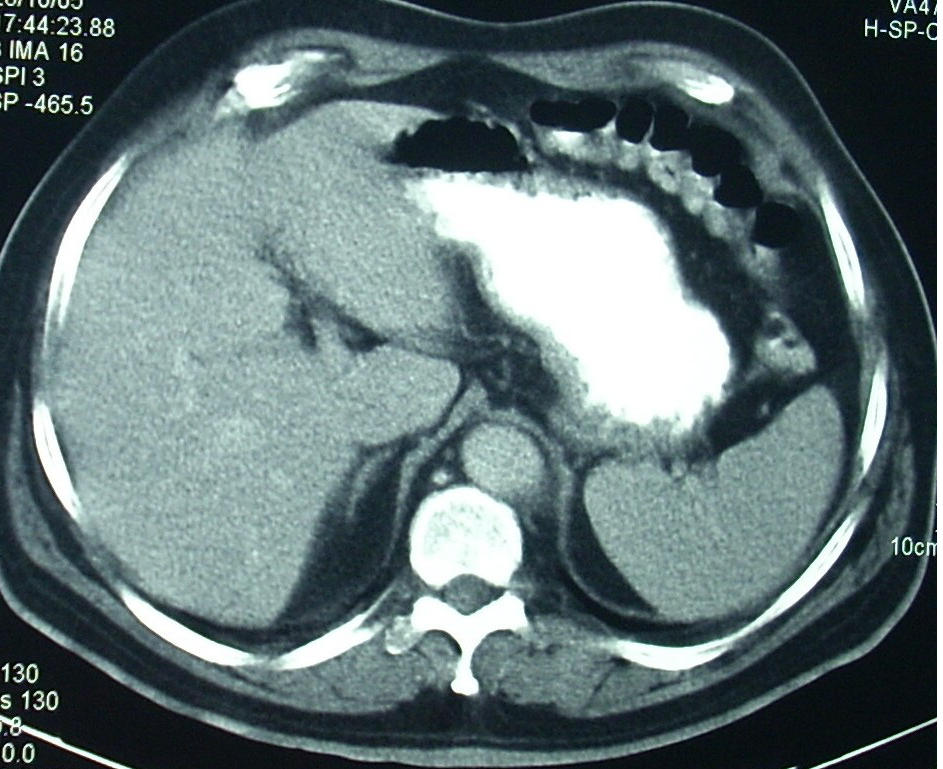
CT at initial diagnosis showing absence of liver involvement.
